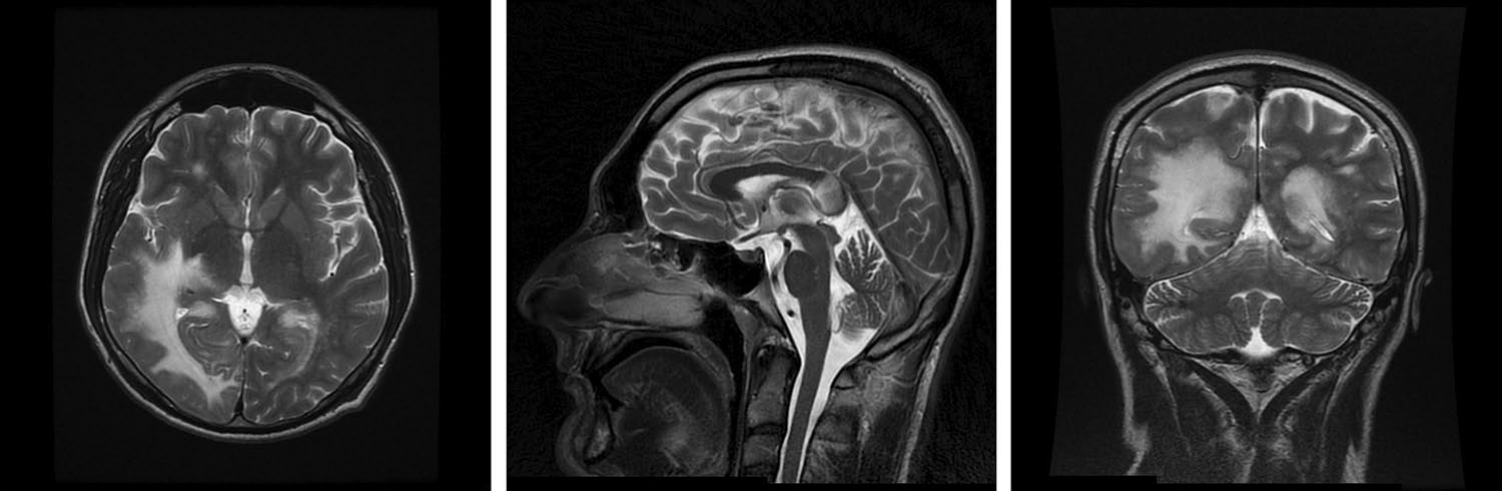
Patient A: T2-weighted brain MRI, transverse, sagittal, and coronal planes
